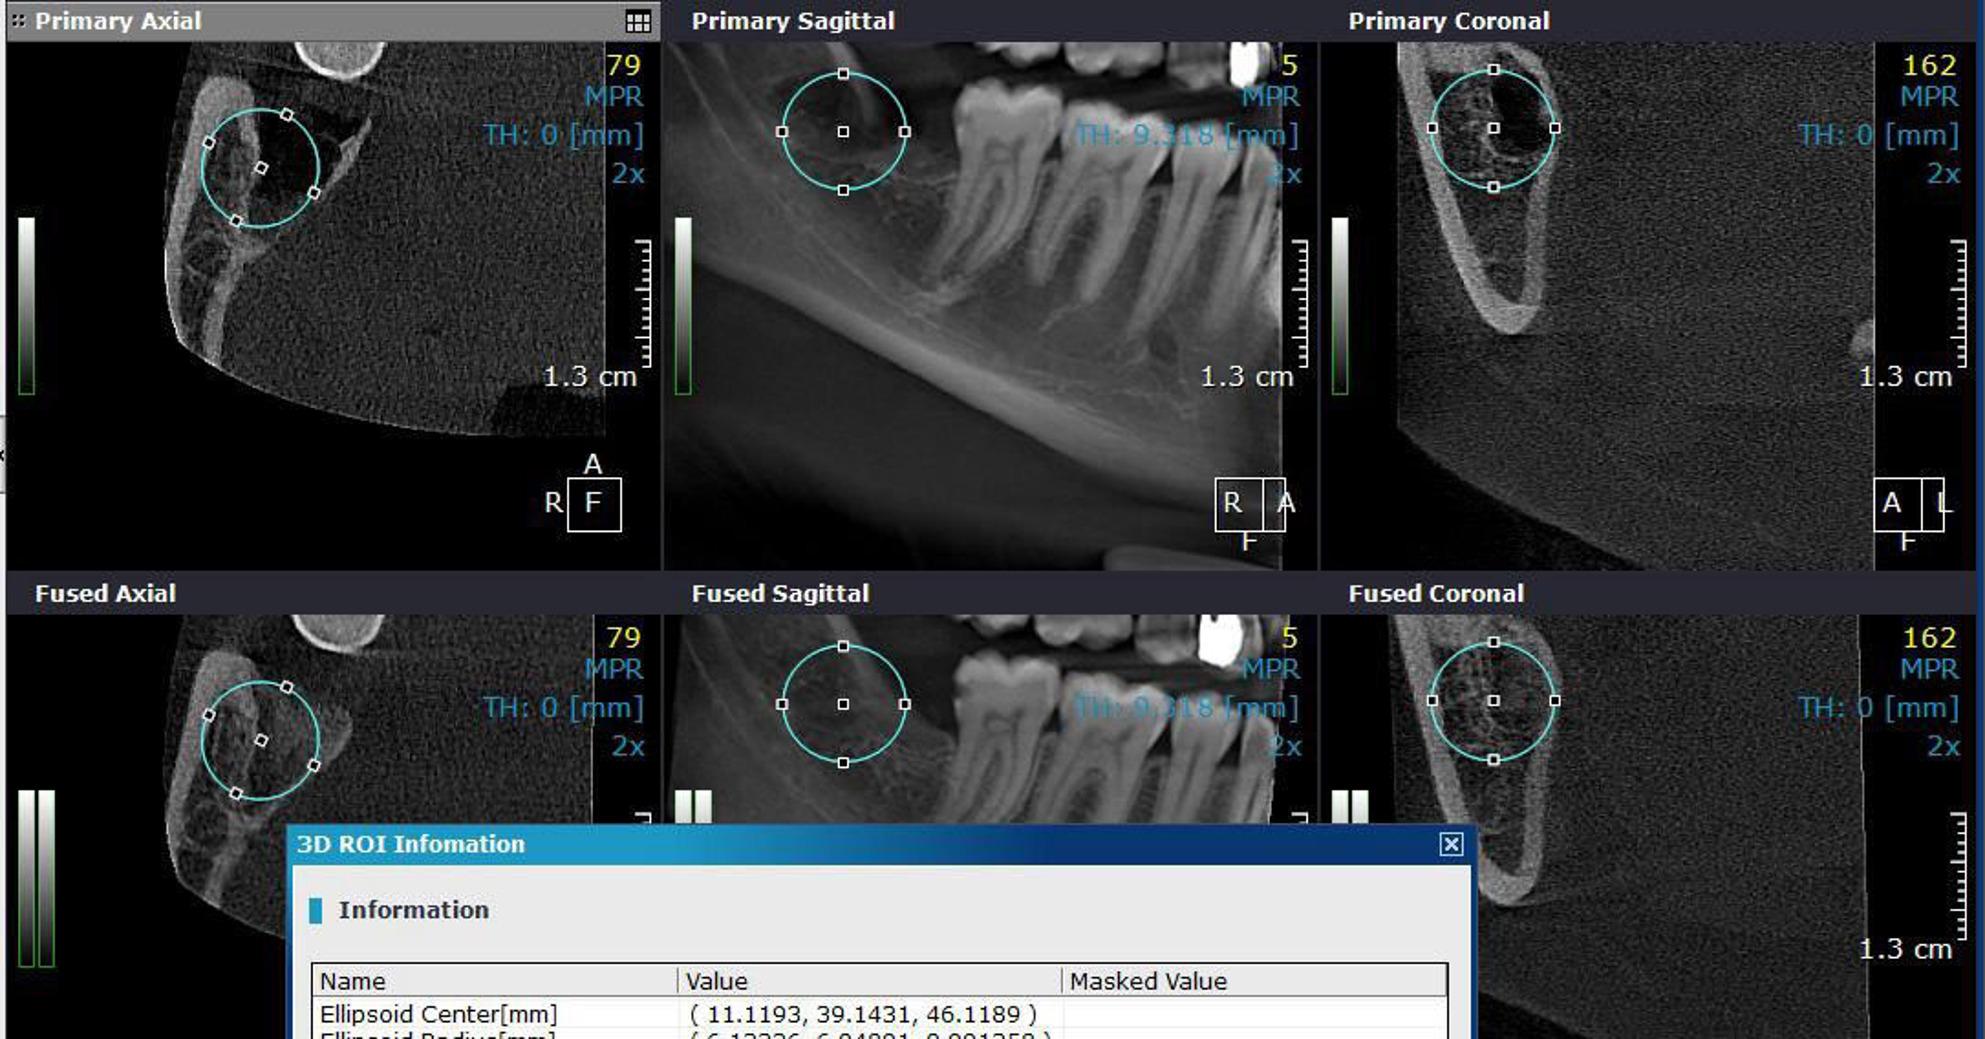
CBCT multiplanar reconstruction images (axial, sagittal, and coronal views) demonstrating the three-dimensional region of interest (ROI) selected within the extraction socket for bone density assessment. The mean gray value and related parameters were calculated using the imaging software
